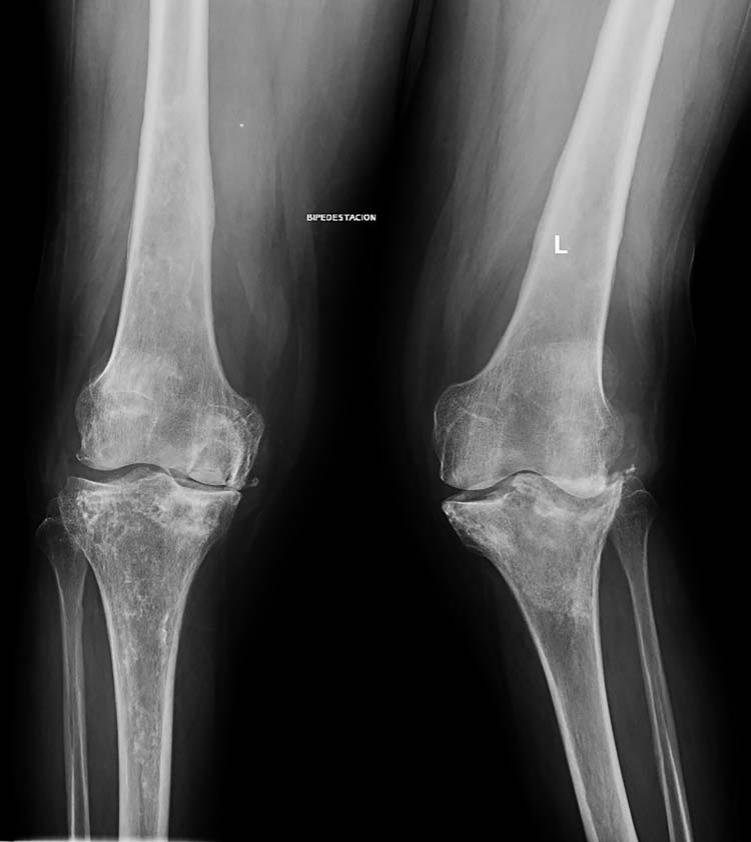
Standing X-rays of the left knee 8 months later showed collapse with depression of the external tibial plateau and associated bone defect that caused 28° valgus deformity.
